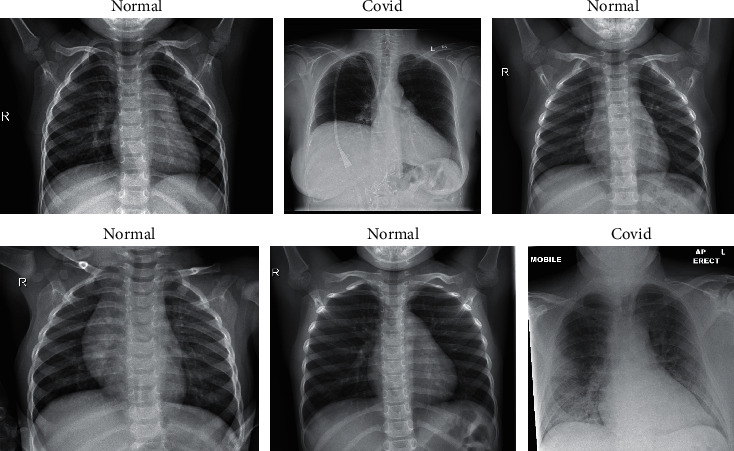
Images random from the COVID-X-ray-5k data set [33].
